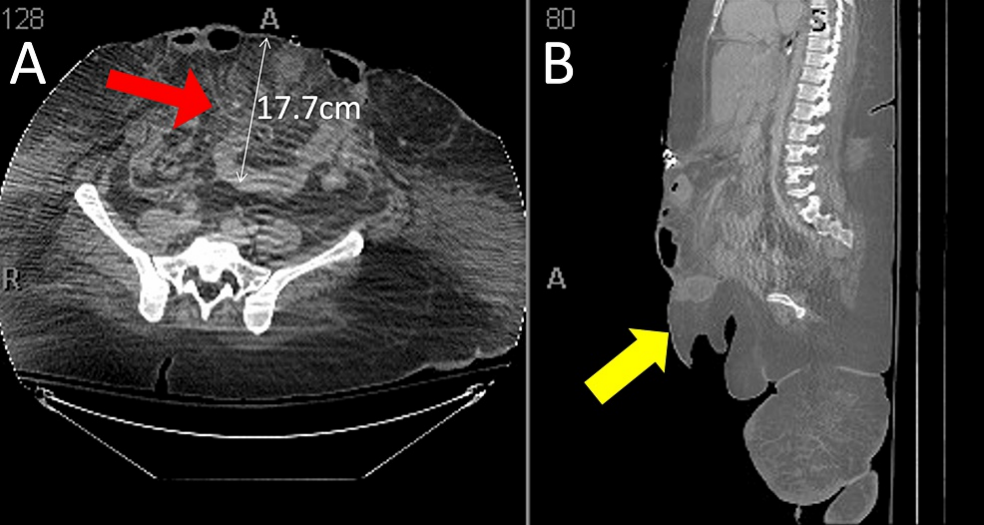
Preoperative computed tomography (CT) scans of the patient's abdomen and bowel herniation. A) Axial CT image of the pelvis showing a 17.7cm deep herniation (red arrow) of the small and large bowel protruding through the muscular abdominal wall and subcutaneous adipose tissue. B) Sagittal CT image showing the bowel herniation (yellow arrow) through the abdominal wall and hanging below the pubic symphysis.
